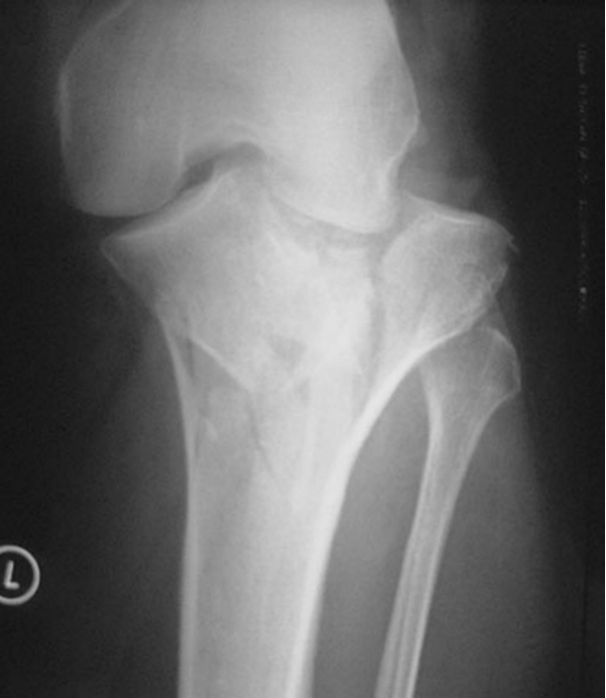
Schatzker type VI fracture, pre-op X-rays (AP)
